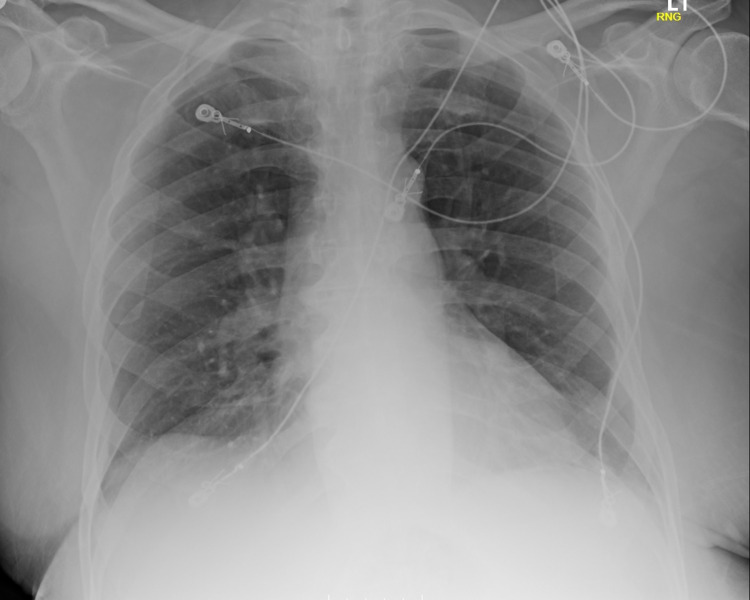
Portable chest X-ray A single anteroposterior chest X-ray was obtained on admission. No acute intrathoracic process was observed. Degenerative change of the spine and shoulders was documented.
